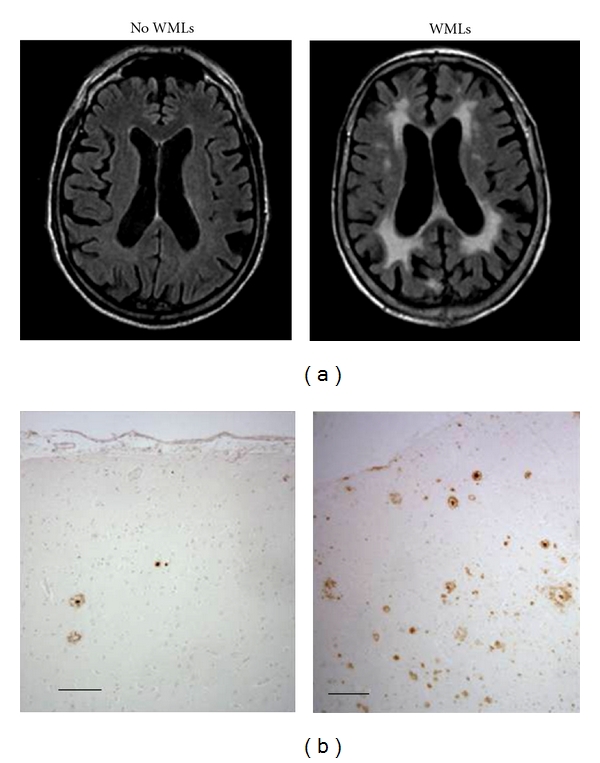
(a) MRI scans with and without white matter lesions and (b) corresponding *β*-amyloid pathology in the cortex. Bar = 100 *μ*m.
